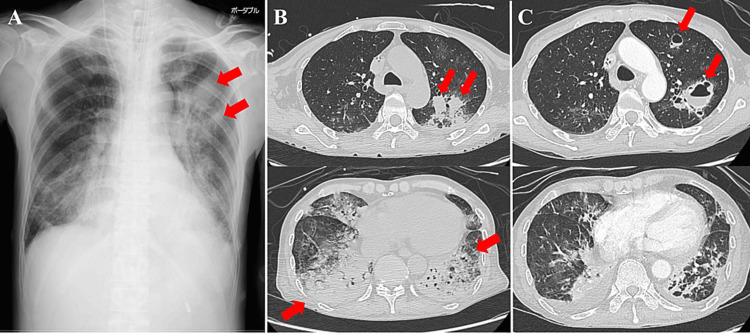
Chest radiography and CT images for case 1. (A) Chest radiography upon admission for the patient in case 1 revealing consolidation in the right lower and entire left lung fields. Two nodules were observed in the left lung fields (red arrows). (B) Chest CT images upon admission for the patient in case 1 revealing extensive consolidation in the right middle and lower lobes and the entire left lobe (red arrows). (C) Contrast-enhanced chest CT images on day 13 for the patient in case 1 revealing several pulmonary abscesses in the left upper lobe (red arrows). Consolidations in the bilateral lower lobes improved.
